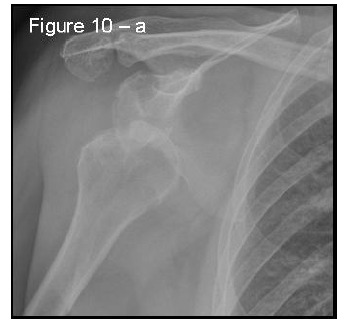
A case of bipolar bone defect on AP X-ray 57x55mm (150 x 150 DPI).
